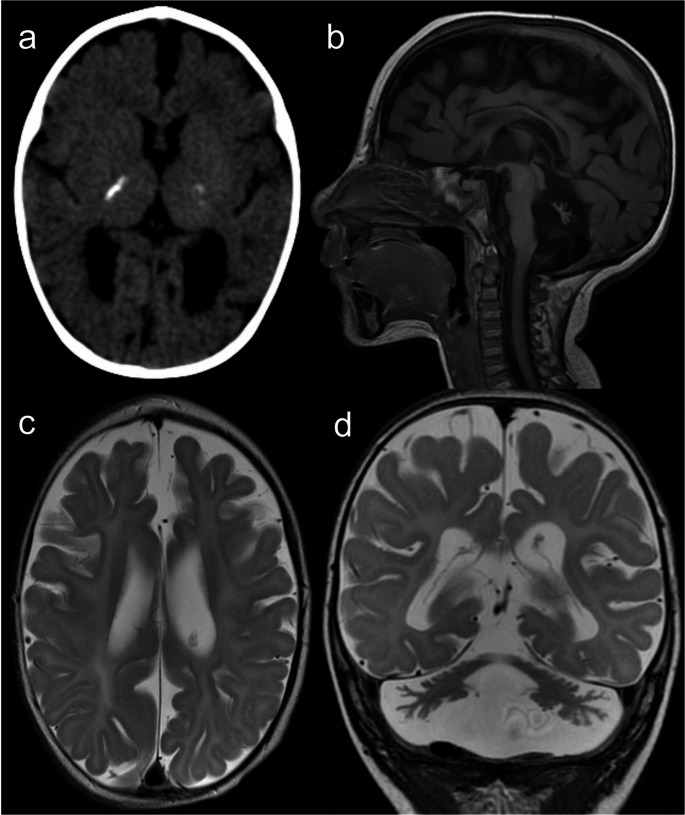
Hoyeraal-Hreidarsson syndrome. An 8-month-old male patient with microcephaly, developmental delay, and oral and skin lesions. Head CT (a) and brain MRI (b – sagittal T1, c – axial T2, and d – coronal T2) scans depict prominent pontocerebellar hypoplasia and coarse calcifications in the internal capsules. Other abnormalities include discrete T2 hyperintensity of the cerebral white matter, hypoplastic corpus callosum, and microcephaly with a simplified gyral pattern. These imaging findings are suggestive of Hoyeraal-Hreidarsson syndrome, confirmed by targeted genetic testing (RTEL1 mutations)
